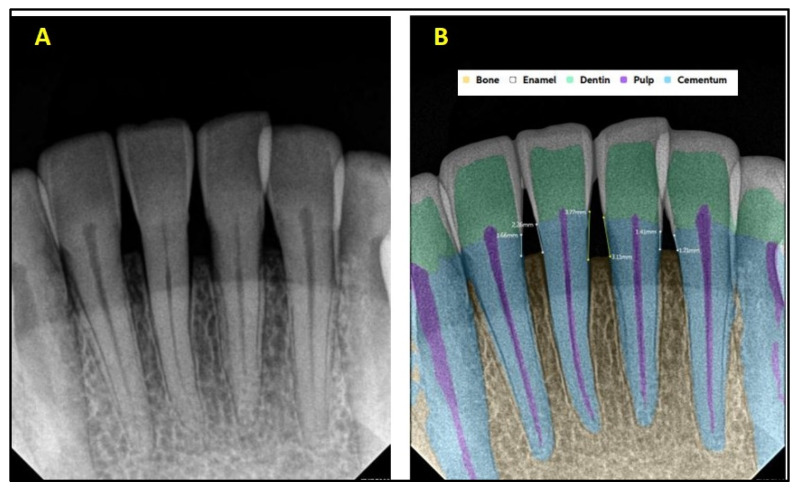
(A) Intraoral periapical radiographic image of mandibular anterior teeth; (B) analysis made by artificial intelligence software depicting bone loss.
